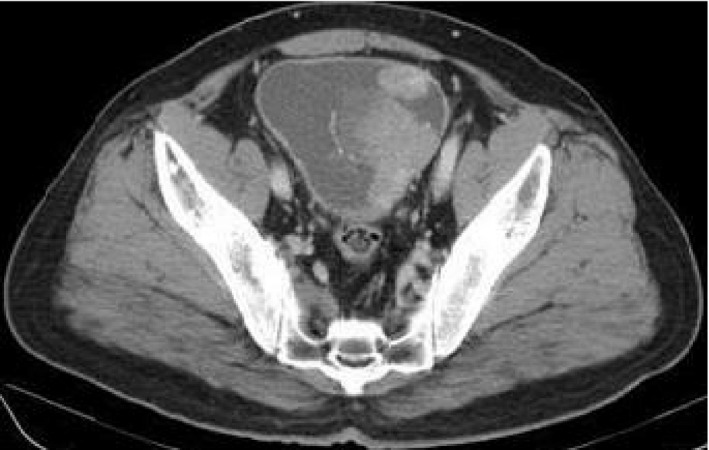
CT scan findings of the muscle‐invasive bladder cancer before RARC.
